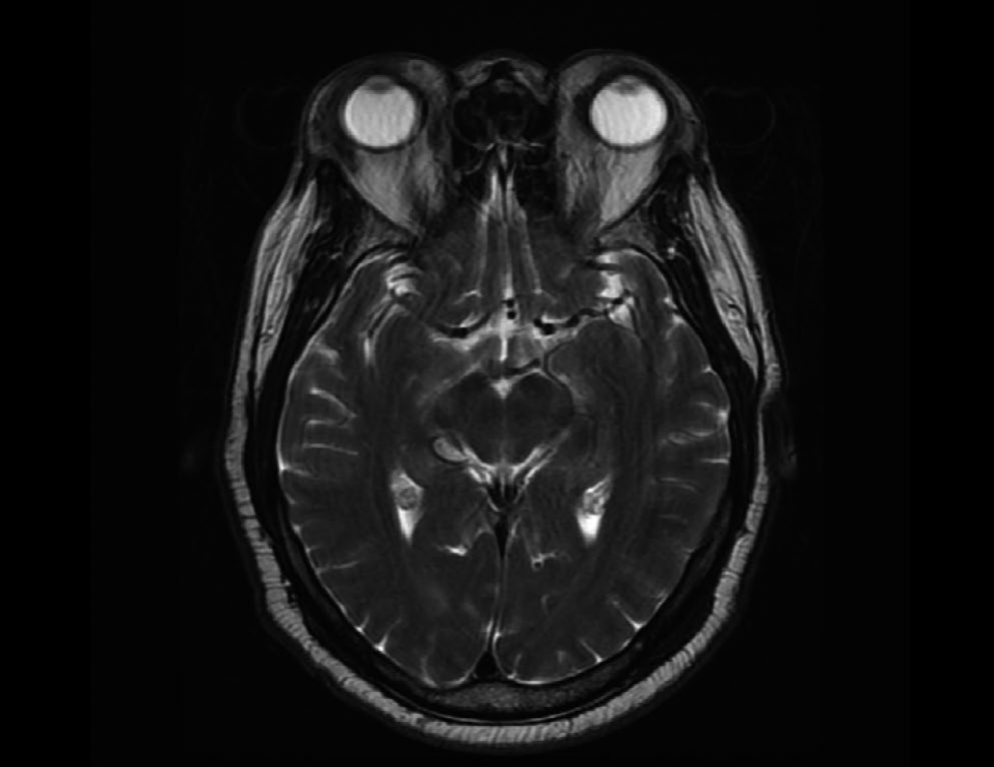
MRI brain/orbit demonstrating bilateral proptosis
